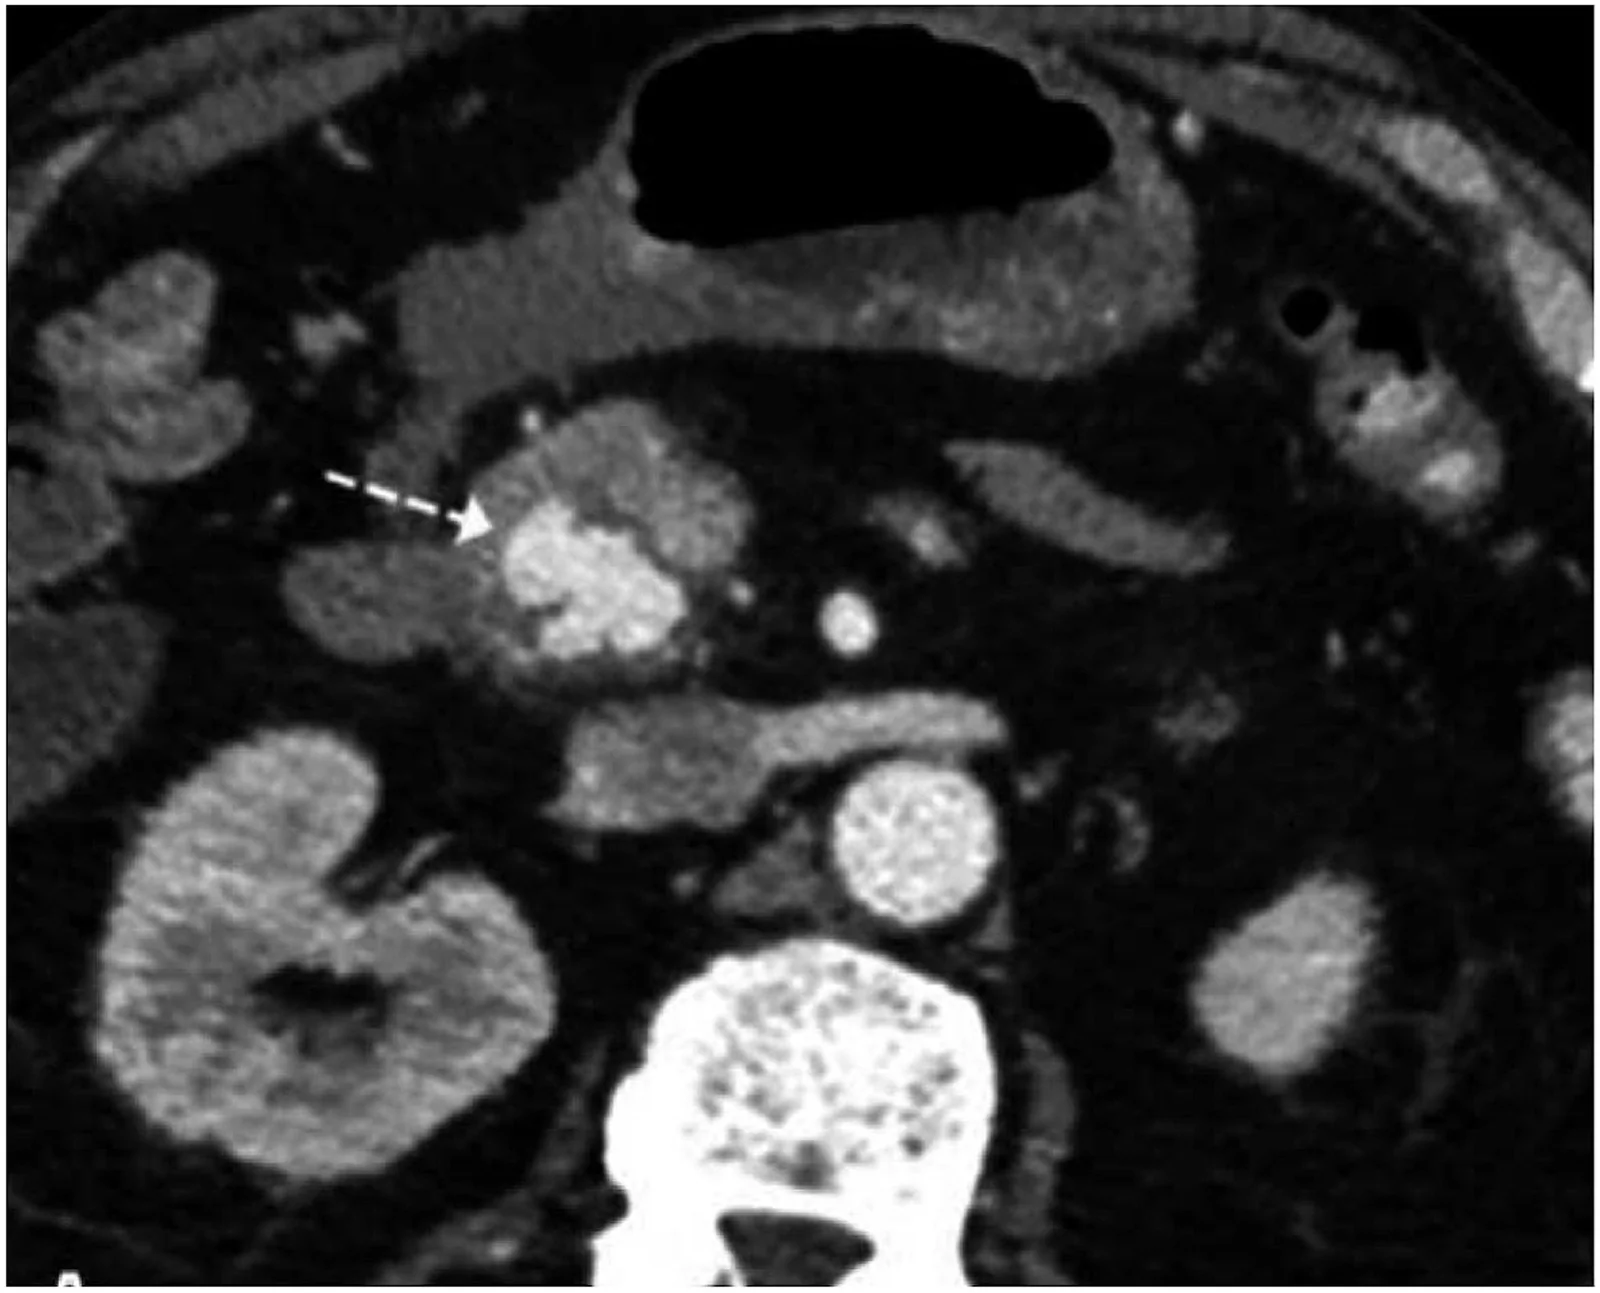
Axial contrast-enhanced CT through the abdomen at the level of the pancreatic head with arrows pointing to a large pseudoaneurysm.
